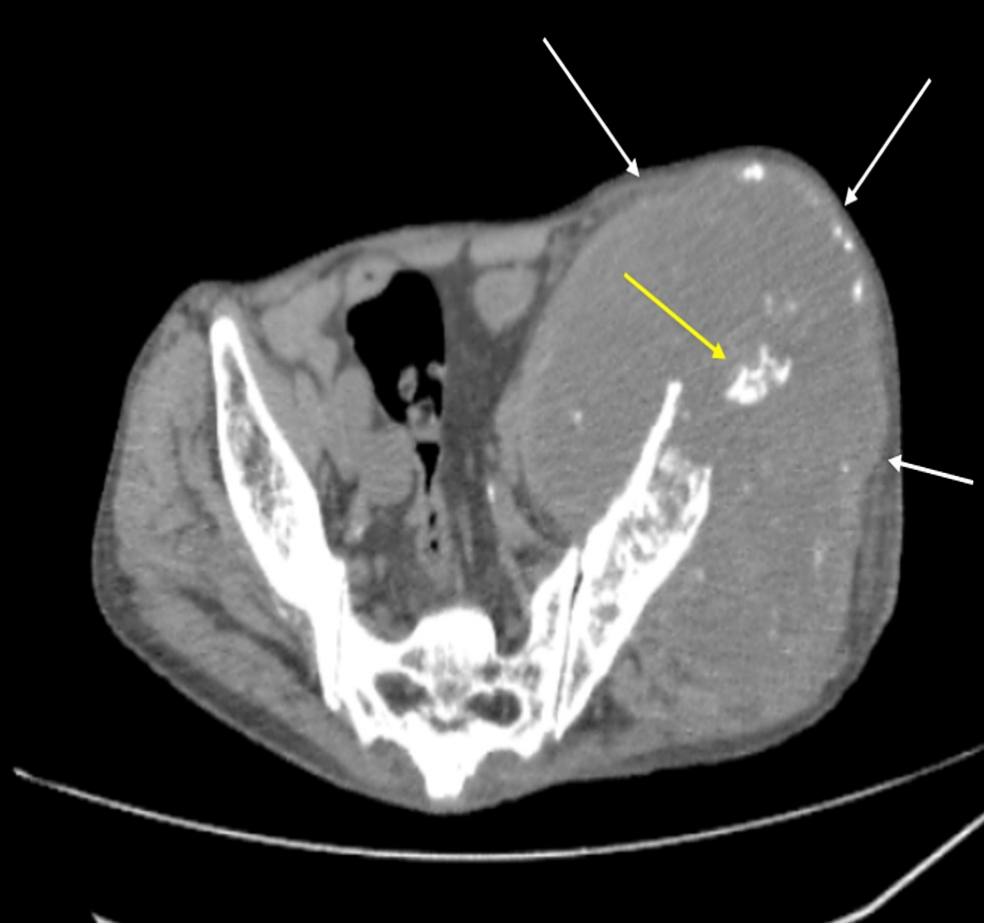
CT showing large large soft tissue mass encasing left iliac blade (white arrows). Few calcific foci are noted in central (yellow arrow) and periphery.
